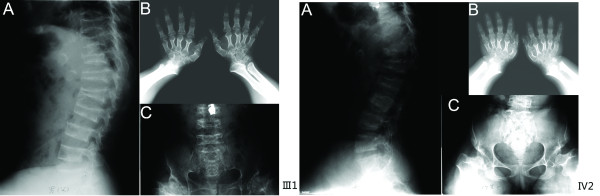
Similar radiographic features for individual III1 and IV2. (A) Anterior beaking of the vertebral bodies and distinctive plantyspondyly, mild lumber lordosis. (B) Short metacarpals and phalanges, small carpal bones, cone-shaped epiphyses, irregular metaphyses of the ulna and radius. (C) Mild scoliosis, small femoral heads, flared metaphyseal borders, poorly developed acetabulae with enlarged acetabular angles, and widened symphysis pubis.
